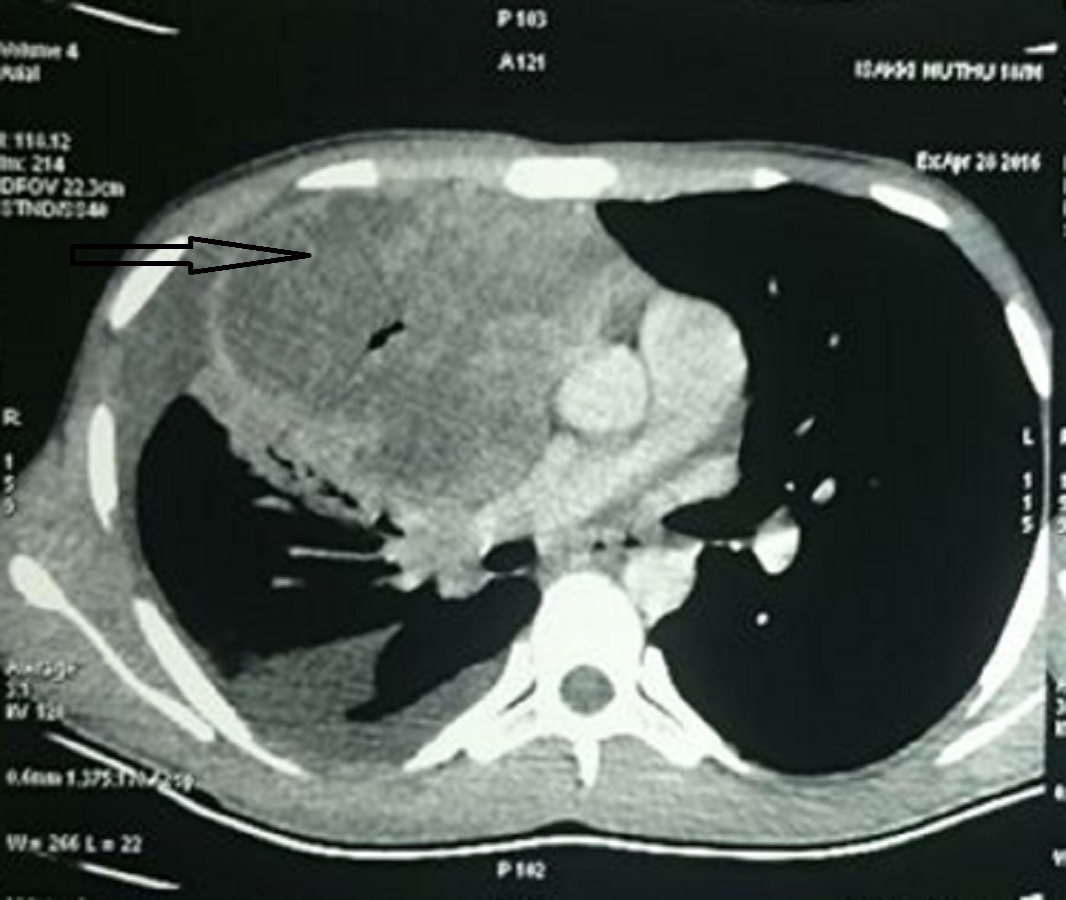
CT of the thorax showing the mass lesion in the upper lobe of the right lung CT - Computed tomography.
